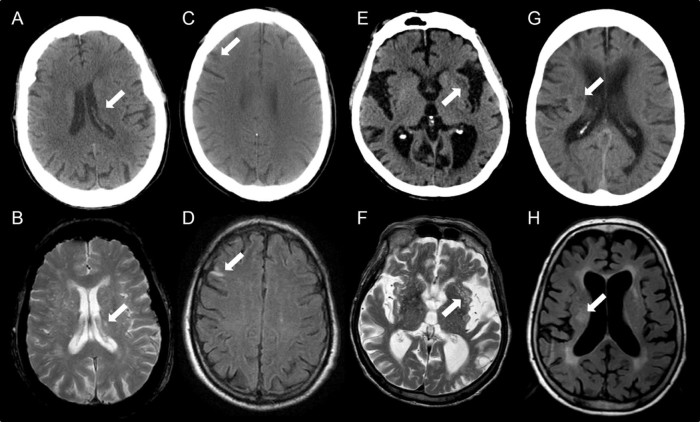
Examples of misclassification of old infarcts between CT and MRI. A small lacunar infarct was just visible on CT (A) but below the size threshold for recording. The same lesion appeared larger on T_2_w MRI (B). A small cortical lesion missed on CT (C) was obvious on FLAIR MRI due to tissue signal changes (D). Low attenuation in the external capsule was recorded as an old infarct on CT (E) but was found to represent a cluster of EPVS on T_2_w MRI (F). A focal area of low attenuation observed on CT was identified as a noncavitated lacunar lesion (G); this was considered to be a WMH on FLAIR MRI (H). All assessments of old infarct agreement was based on data from 1 rater.
